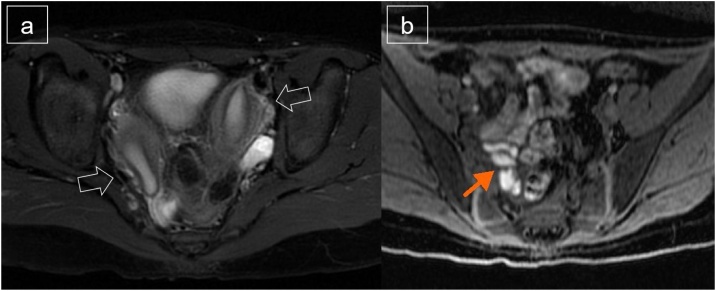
These Figures shows magnetic resonance imaging (MRI) findings. Uterine malformation (a: white arrows) and right hematosalpinx (b: orange arrow) was confirmed with this MRI.
